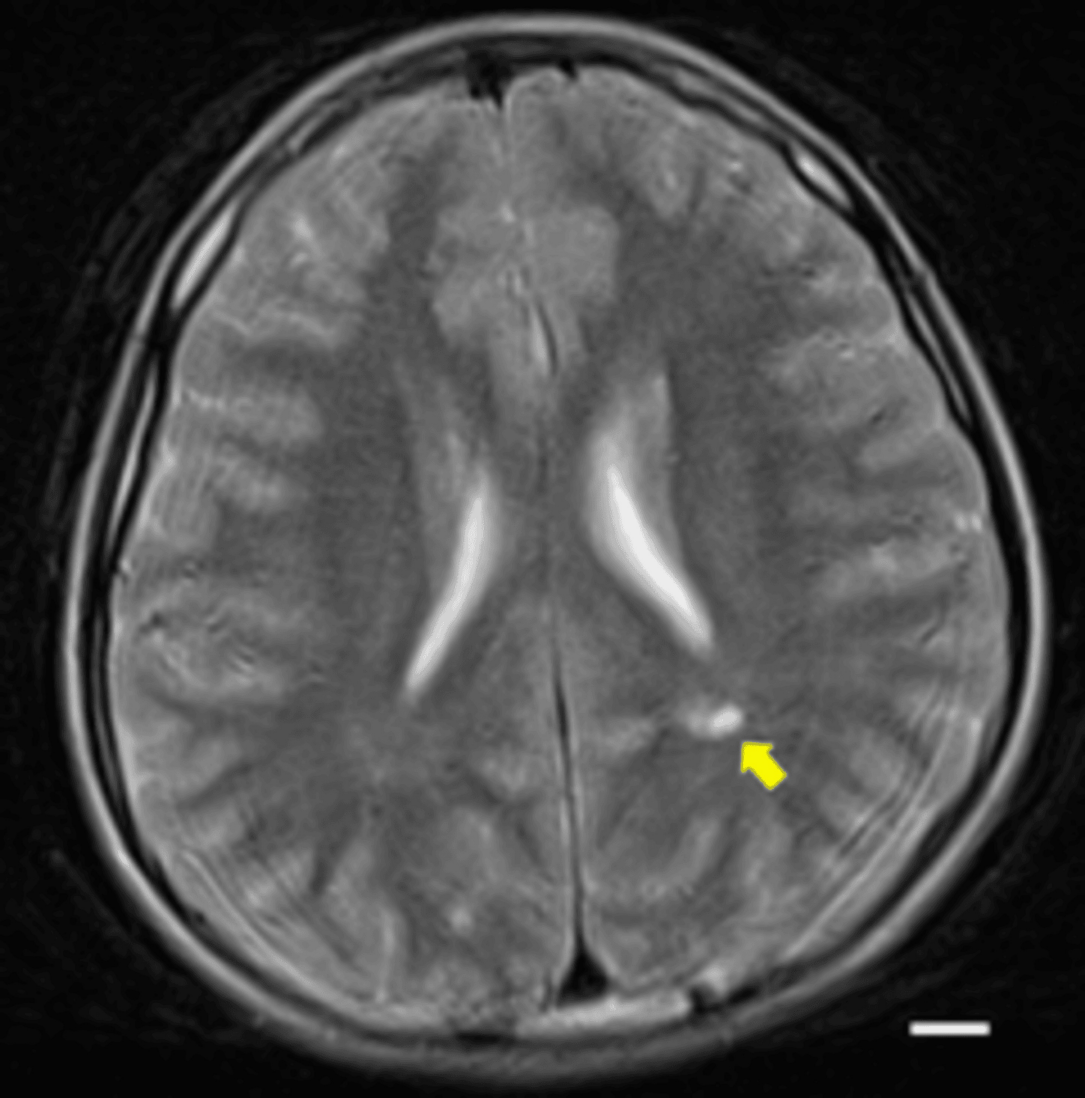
Brain MRI at 11 years and 10 months of age, performed on a 1.5 Tesla scanner. Axial T2-weighted images (slice thickness 5 mm) show a cystic lesion in the deep white matter of the left parietal region near the lateral ventricular trigone (arrow). The lesion measured approximately 10 mm in maximal diameter at both four years and eight months and 11 years 10 months, indicating no significant interval change. No progression of punctate hyperintense areas is observed. Diffusion-weighted imaging (DWI) and apparent diffusion coefficient (ADC) maps showed no restricted diffusion, and susceptibility-weighted imaging (SWI) revealed no hemorrhagic or calcific lesions. A white scale bar indicates 10 mm.
